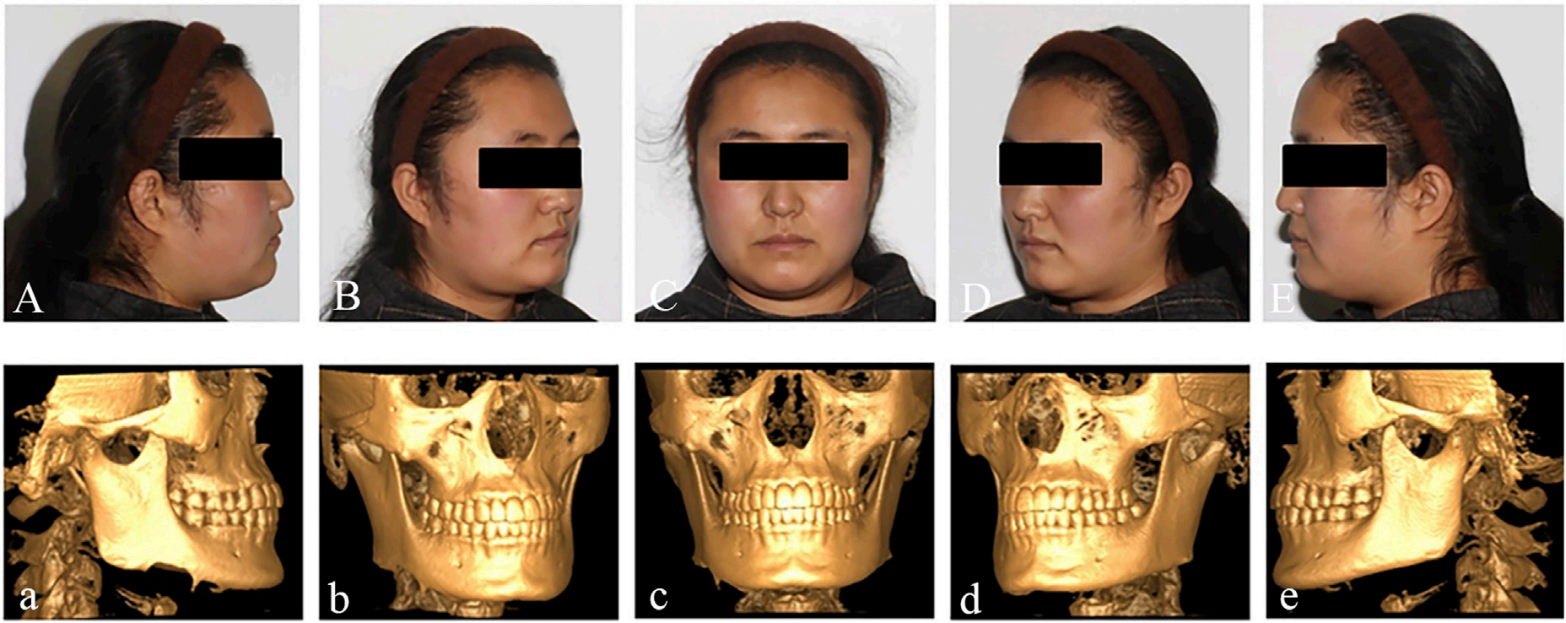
Preoperative facial photos and CBCT.
